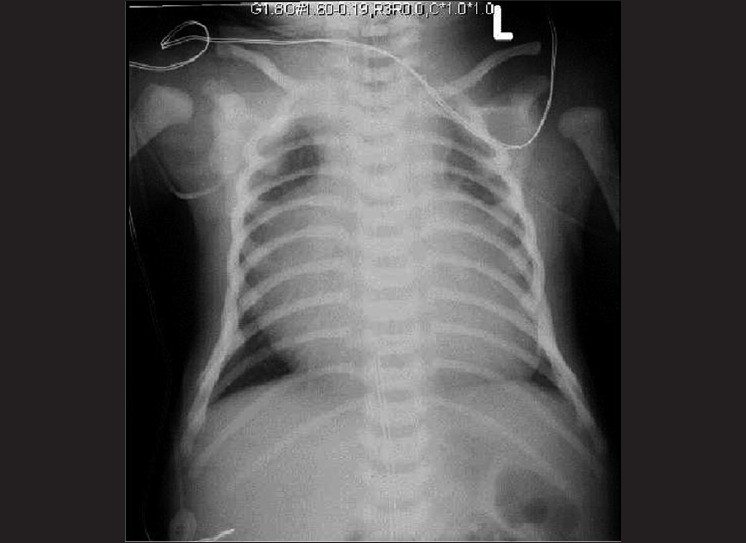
Chest X-ray demonstrating massive cardiomegaly with oligemic appearing lung fields
